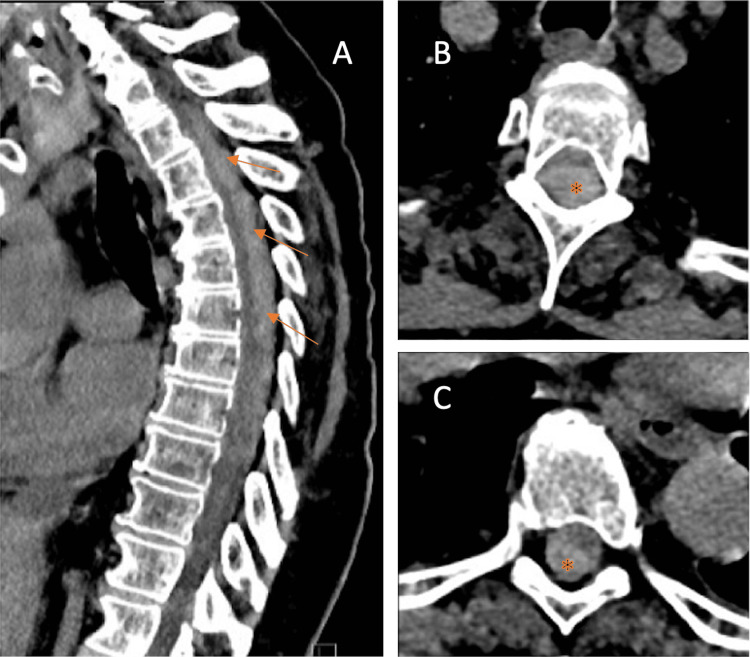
Computed tomography (CT) of the thoracic spine in the first case at presentation, demonstrating an epidural hematoma (arrows and asterisks) extending from T1 to T9. (A) Sagittal image; (B) axial image at T3; (C) axial image at T7.
